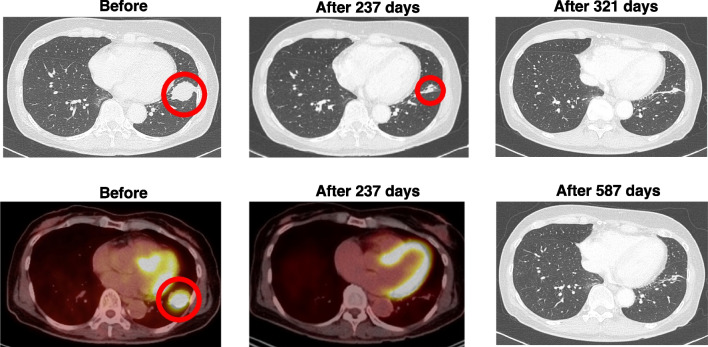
Lung cancer assessed by CT and PET/CT. The patient was diagnosed with lung cancer (35 mm × 30 mm) by computed tomography (CT) and fluorodeoxyglucose (FDG)-positron emission tomography/CT (PET/CT). The tumor size decreased significantly to 12 mm, a 65.7% reduction, after combined therapy with eight doses of WT1/MUC1-DC and erlotinib for 237 days based on CT and FDG uptake by PET/CT. After 321 days of combination therapy, no tumor was detected based on CT. Validation of the tumor’s disappearance persisted for at least 587 days after treatment initiation, without any indication of recurrence or metastasis. Red circles show lung cancer
